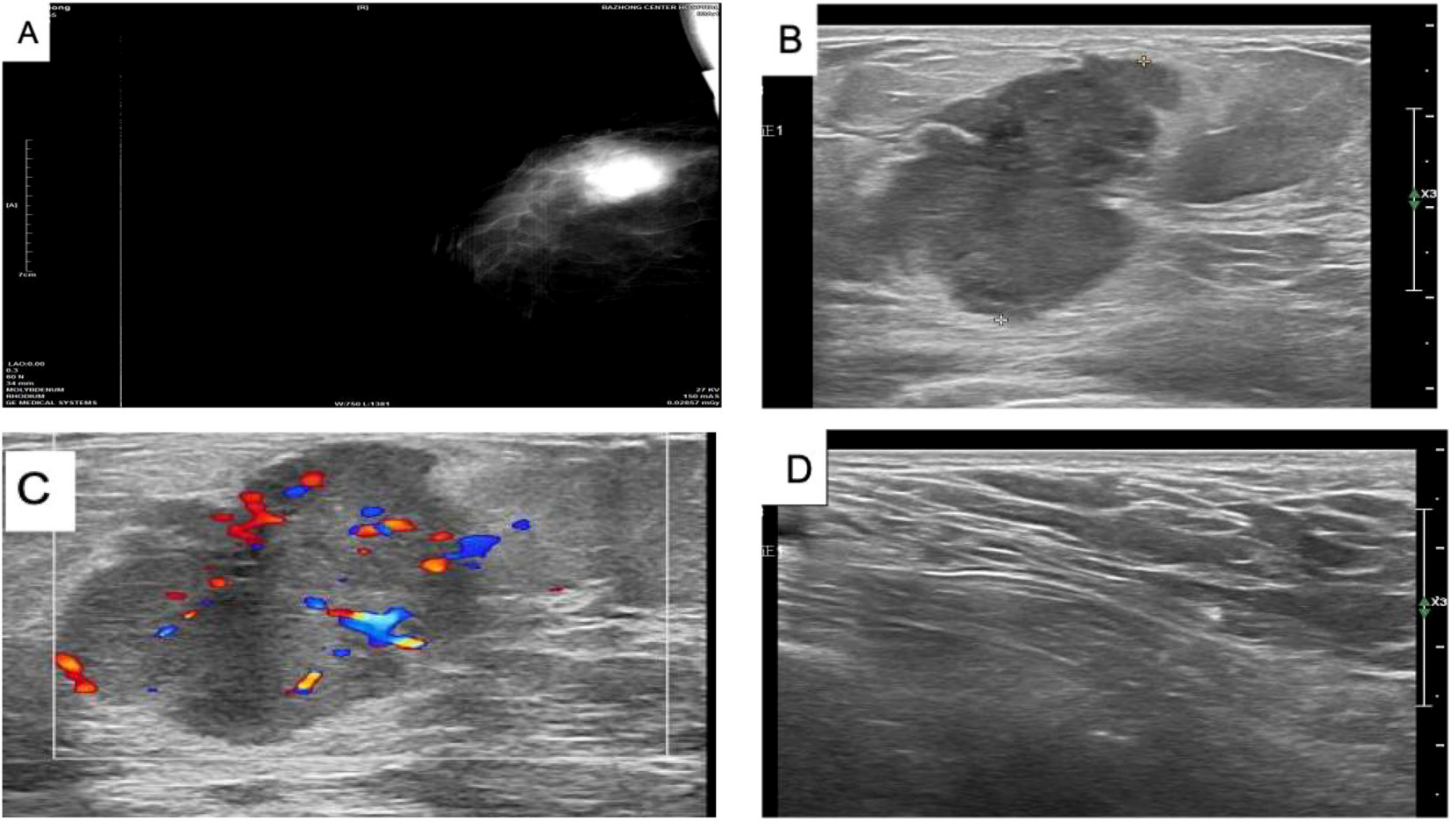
(A) Mammogram of the right breast showing a lobulated mass in the upper quadrant, approximately 3.8 cm × 2.5 cm in size, with irregular margins and a burr sign. No obvious calcification, BI-RADS 4B, and no enlarged lymph nodes in the axilla. (B) Color ultrasound showing a weakly echogenic nodule measuring approximately 28.4 mm × 27.4 mm × 31.2 mm in the upper quadrant of the right breast, with clear borders, irregular morphology, and a few irregular echoes. The nodule appeared lobulated, with a longitudinal/transversal ratio of more than 1, BI-RADS 4b. (C) Color ultrasound showing abundant blood flow detected within the CDEF of the right breast mass. (D) Color ultrasound showing no obvious enlarged lymph nodes in the right axilla.
